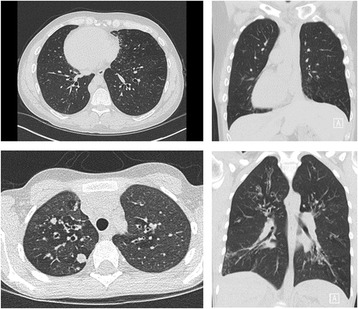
CT images showing dextrocardia and middle lobe abnormalities in adolescent patients with PCD (above), contrasting to upper lobe disease in CF (below)
